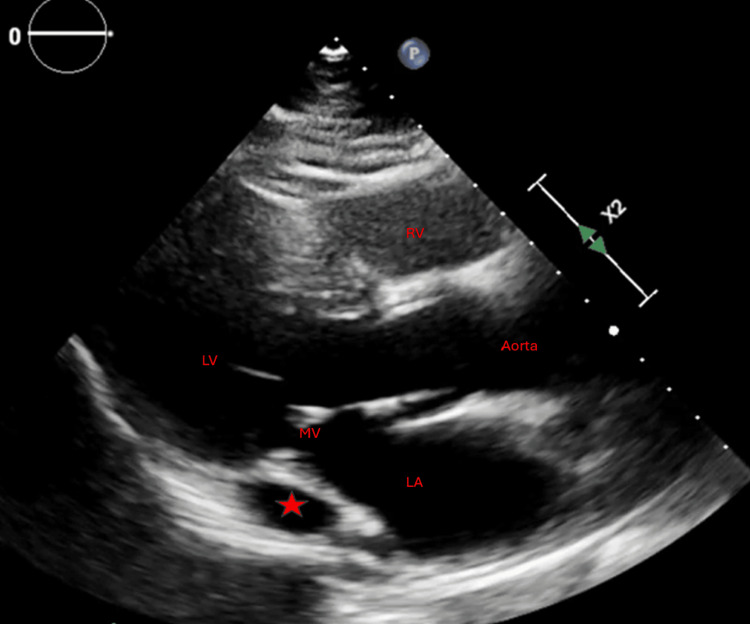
A transthoracic echocardiogram in parasternal long-axis view showing a dilated coronary sinus (red star) The parasternal long axis (PLAX) view is achieved by placing a phased array transducer in the third or fourth intercostal space just to the left of the sternum, with the probe marker directed toward the patient's right shoulder. LA: left atrium; LV: left ventricle; MV: mitral valve; RV: right ventricle
